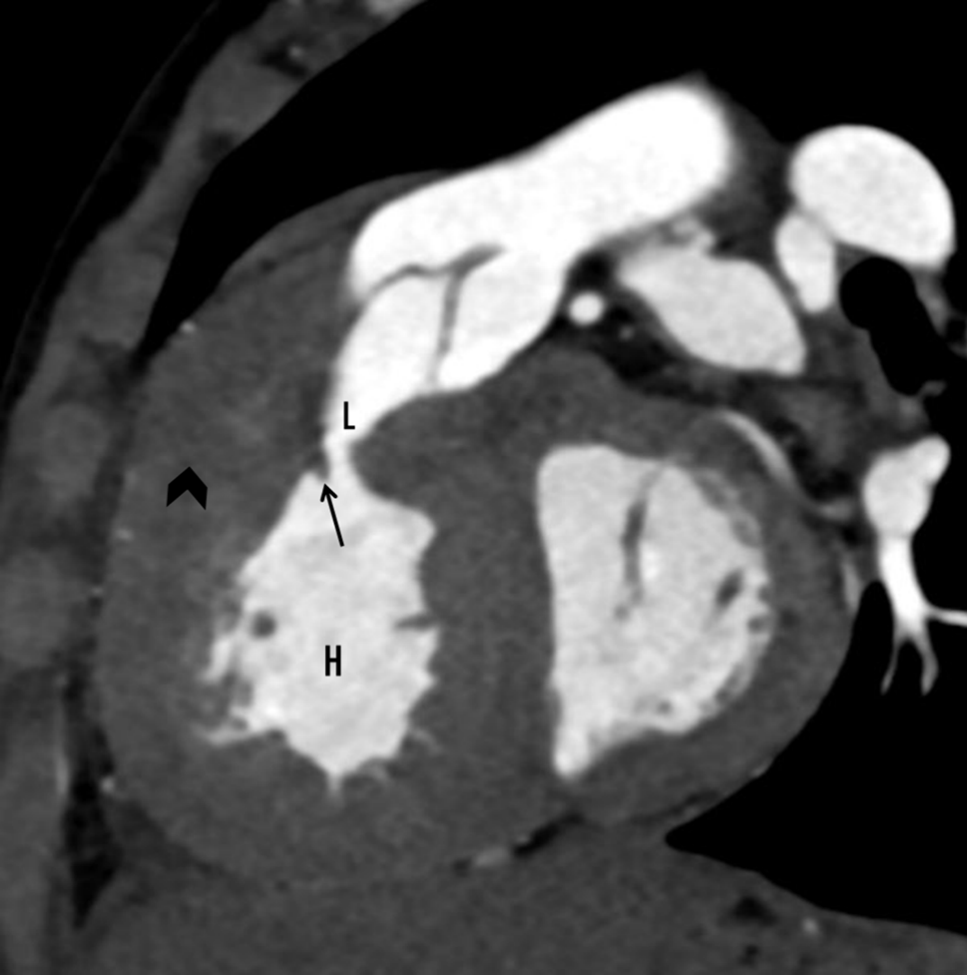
Cardiac CT sagittal section showing a thickened right ventricular wall and infundibulum (chevron) with an anomalous band (thin arrow) running beneath the infundibulum of the outflow tract, which divides the ventricle into high (H) and low (L) pressure chambers
